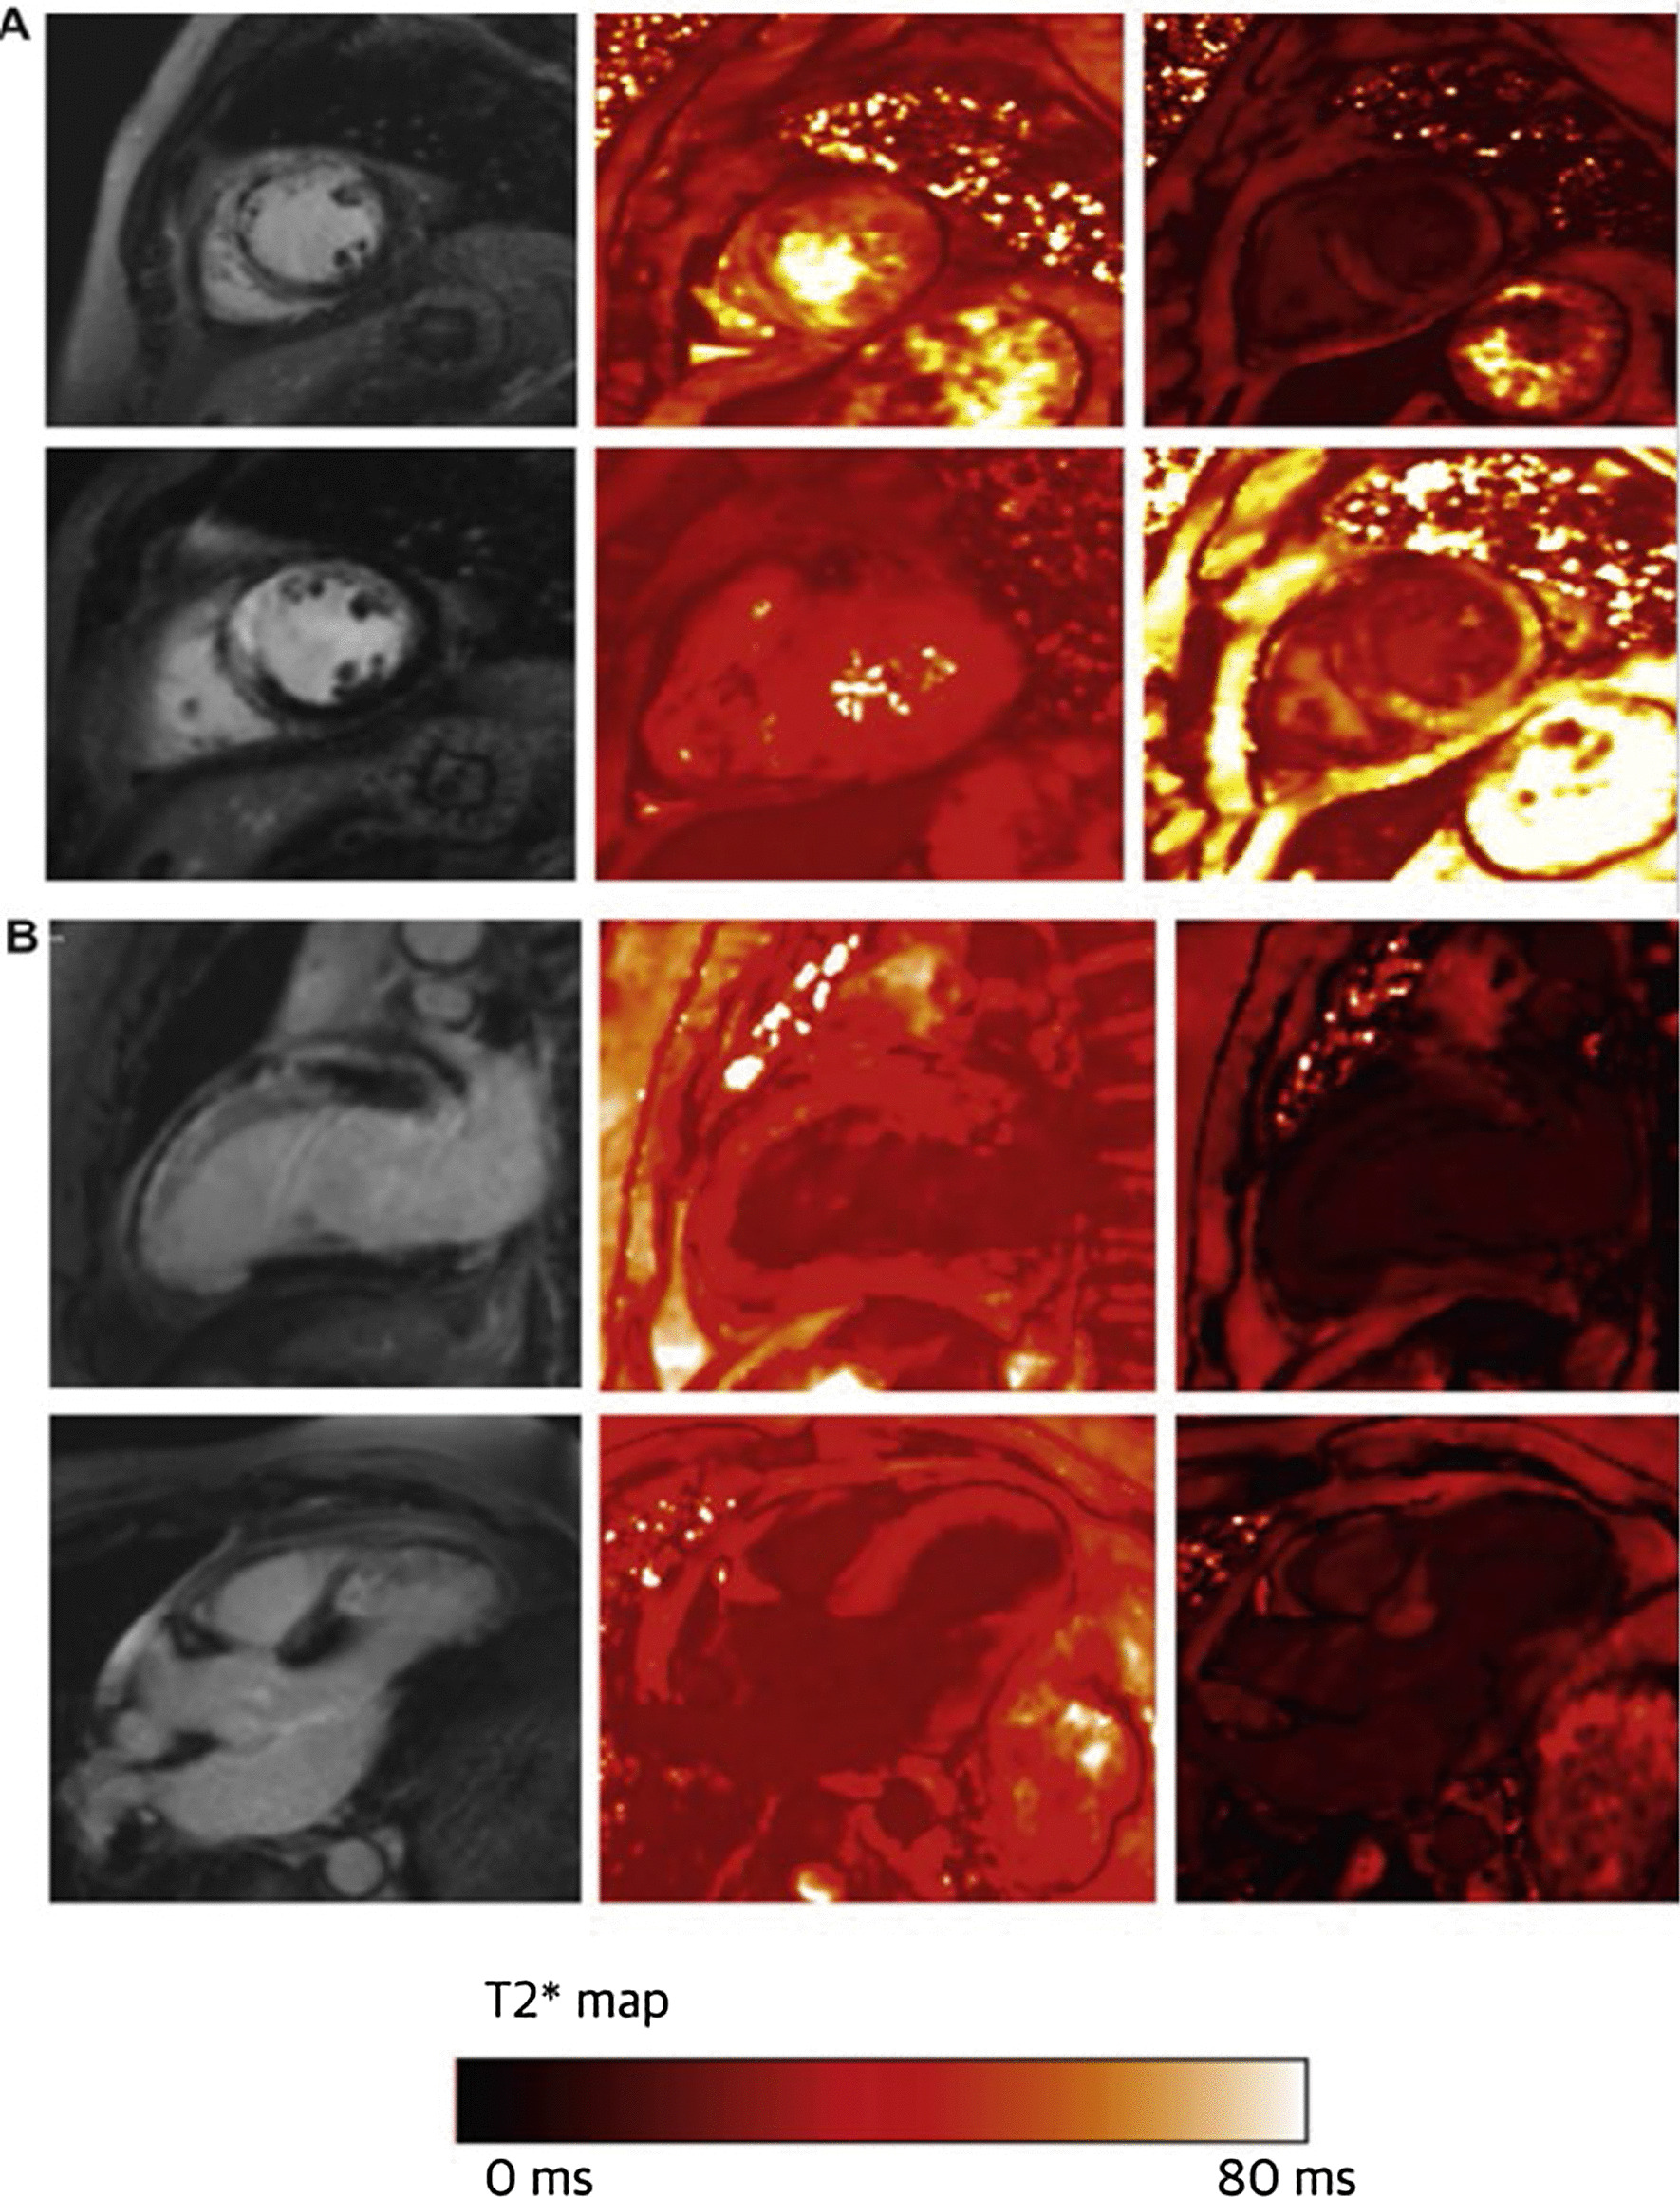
a Patient 1 week post left anterior descending artery infarction with extensive, anterior wall, transmural, mid-ventricle late gadolinium enhancement (LGE) on T1-weighted images (left column), homogeneous myocardial T2 ∗ values before erumoxytol (middle column), but intense dark ferumoxytol uptake in the region of the infarction 24 h post ferumoxytol infusion (right column). b Same patient with anteroapical, transmural LGE, again homogeneous T2 ∗ myocardial values at base, but clear ferumoxytol uptake on T2 ∗ scanning in the region of the LGE 24 h after ferumoxytol infusion. Image reproduced with permission from Merinopoulos et al. [10]
